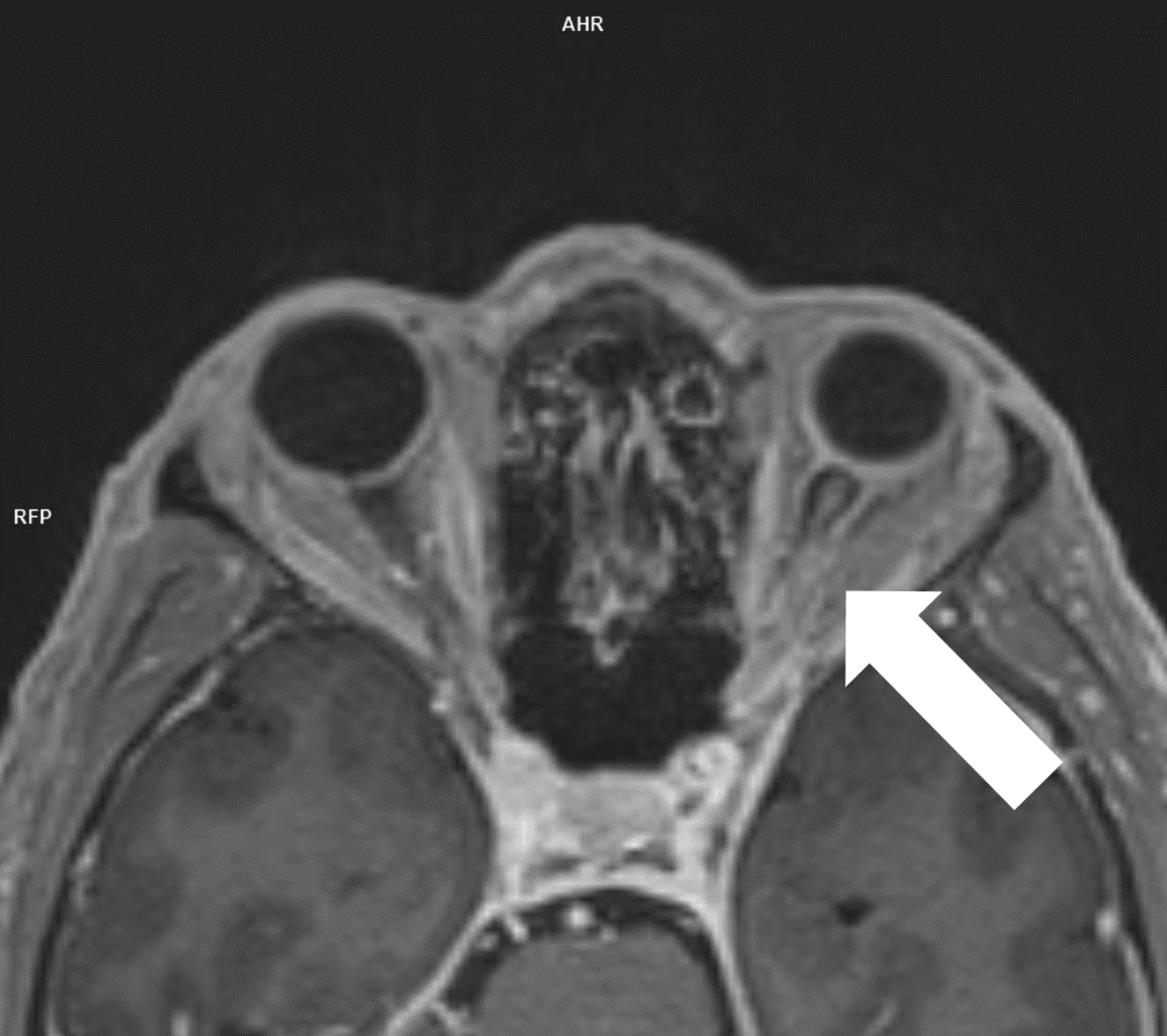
MRI head: transverse contrast-enhanced T1-weighted sequence. White arrow: Patchy enhancement of the left optic nerve on the transverse T1-weighted contrast-enhanced imaging.
